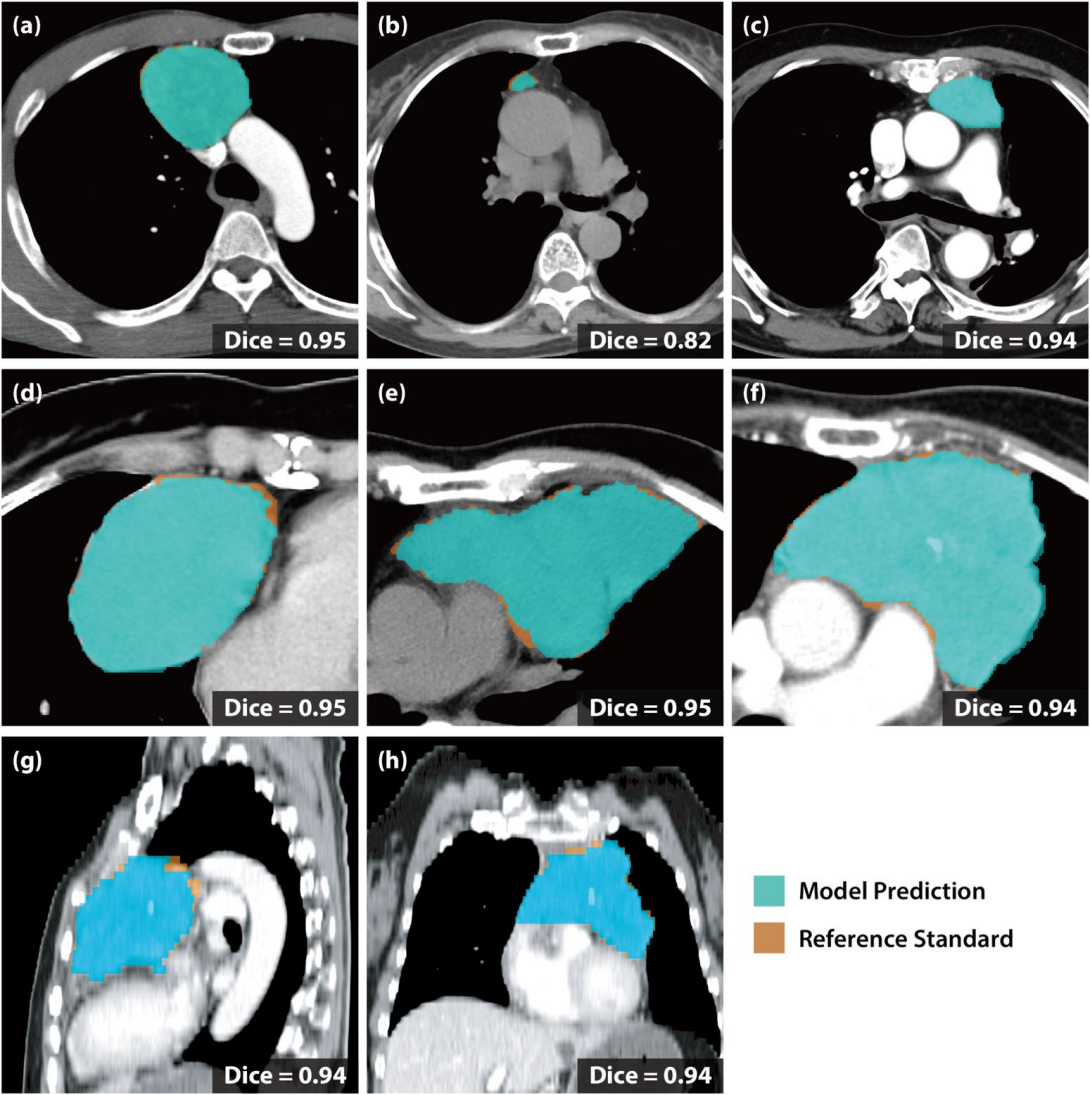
Per-case qualitative analysis of successful cases. Six representative CT images with Dice scores above 0.70 show that the model prediction (shown by the blue regions) closely matched the manual annotations (shown by the orange regions), indicating accurate tumor delineation (a–f). (g) and (h) display the sagittal and coronal views of (f), respectively.
